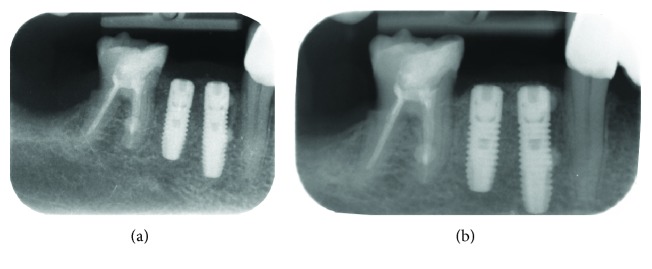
Radiographic exams. (a) Rx right after implant surgery: it highlights the correct implant axis, the connection with the proximal roots, and the implant's fitness under the bone crest. (b) Rx control after 6 months: it shows the physiological bone remodeling around the implant's head.
